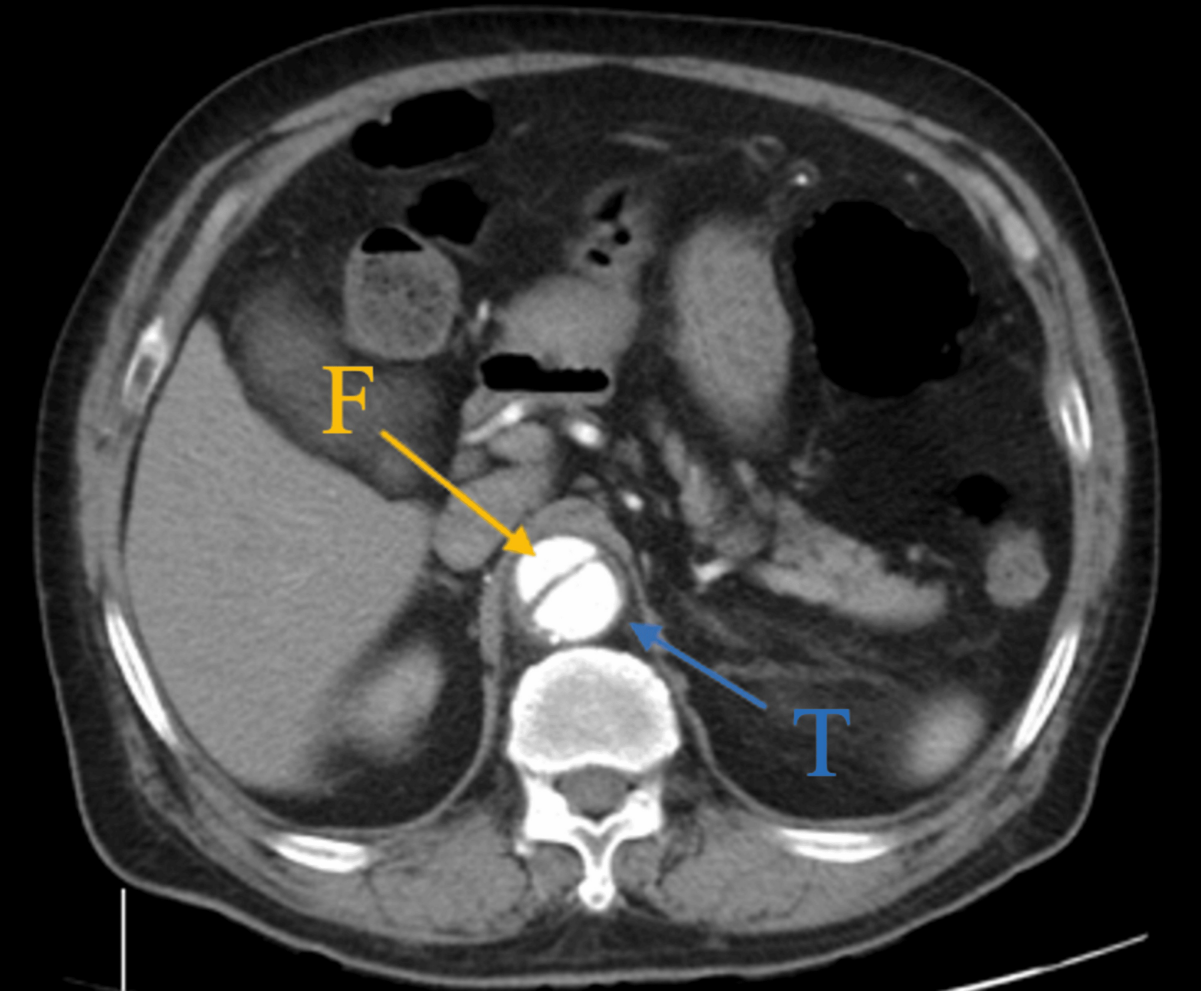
Abdominal computed tomography (CT) scan with contrast enhancement in axial view The abdominal CT shows aortic dissection with an intimal flap noted in the thoracic aorta (the larger true lumen and smaller false lumen were, respectively, marked with blue and yellow arrows).
